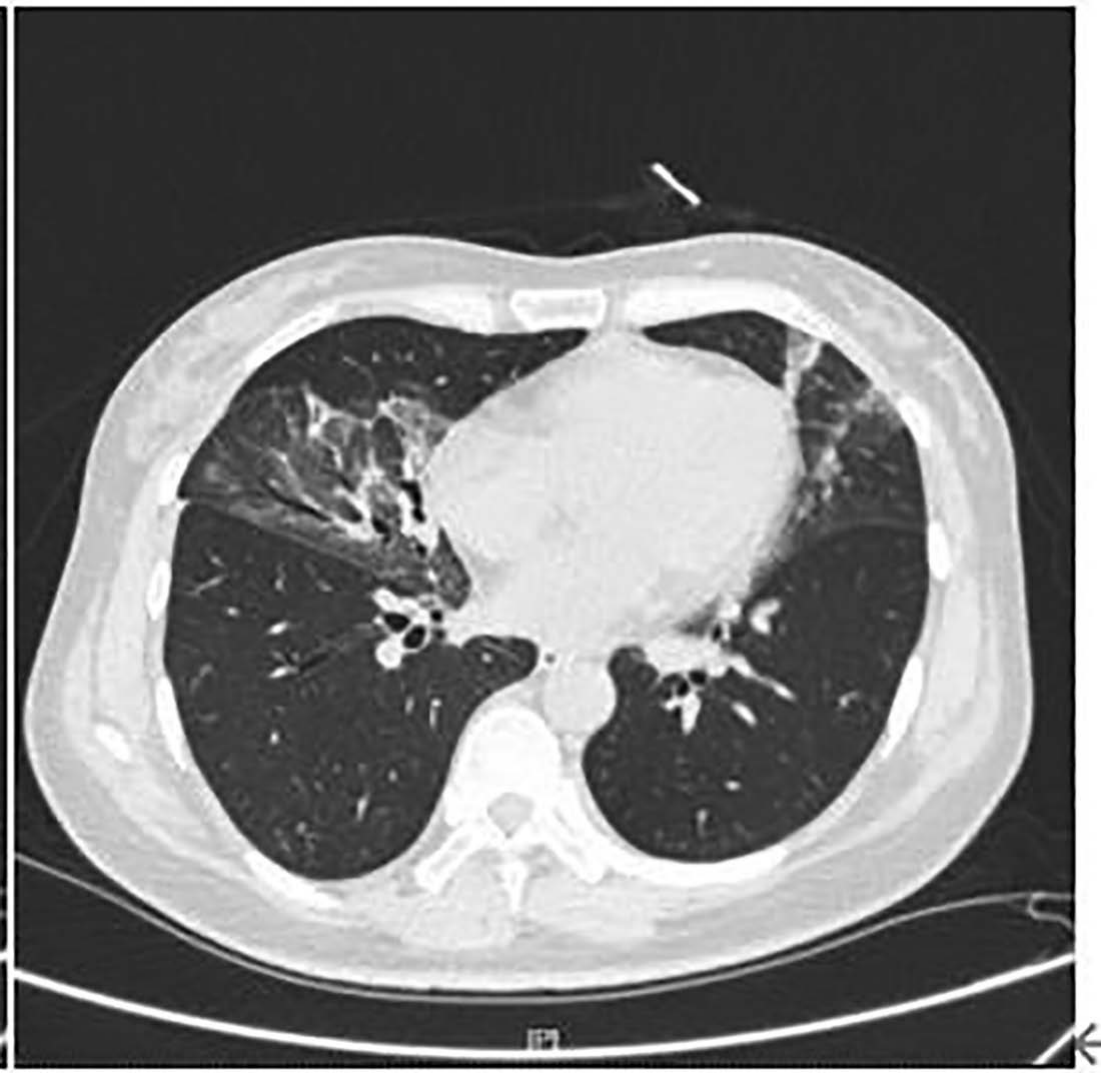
Patient’s pre-admission lung CT on October 12, 2023. CT = computed tomography.
